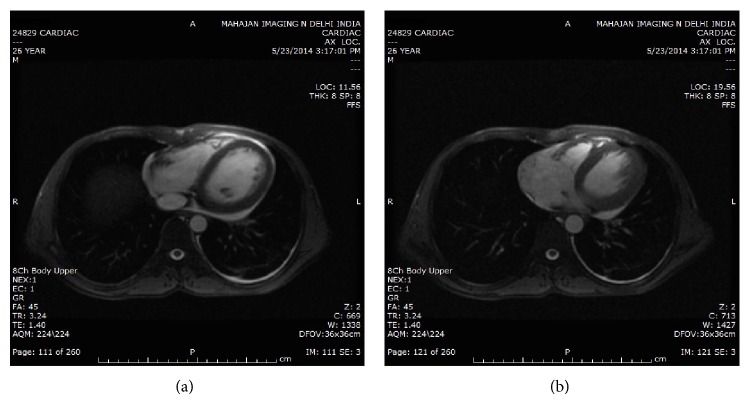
(a) and (b) Cardiac MRI of patient 1.
